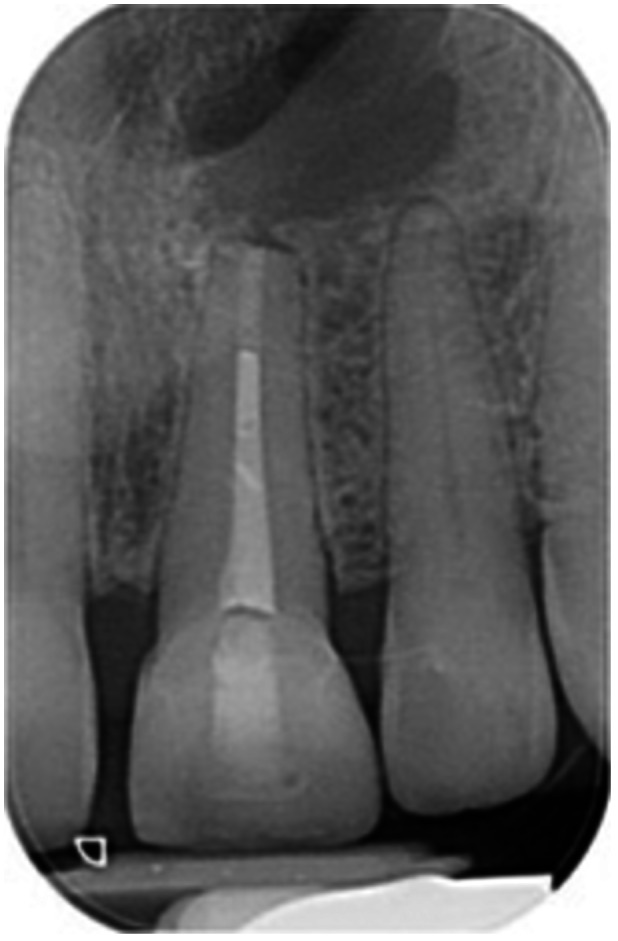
Case 5: The preoperative CBCT and periapical radiograph show an extensive periapical radiolucency associated with tooth 21, which has extended to teeth 22 and 23. Apical surgery was performed on tooth 21 after the completion of a satisfactory nonsurgical root canal retreatment on the tooth. At 6 months, marked healing can be seen, evidenced by a reduction in the periapical radiolucency size associated with tooth 21, with PDL reattachment noted around the roots of teeth 21 and 22.
